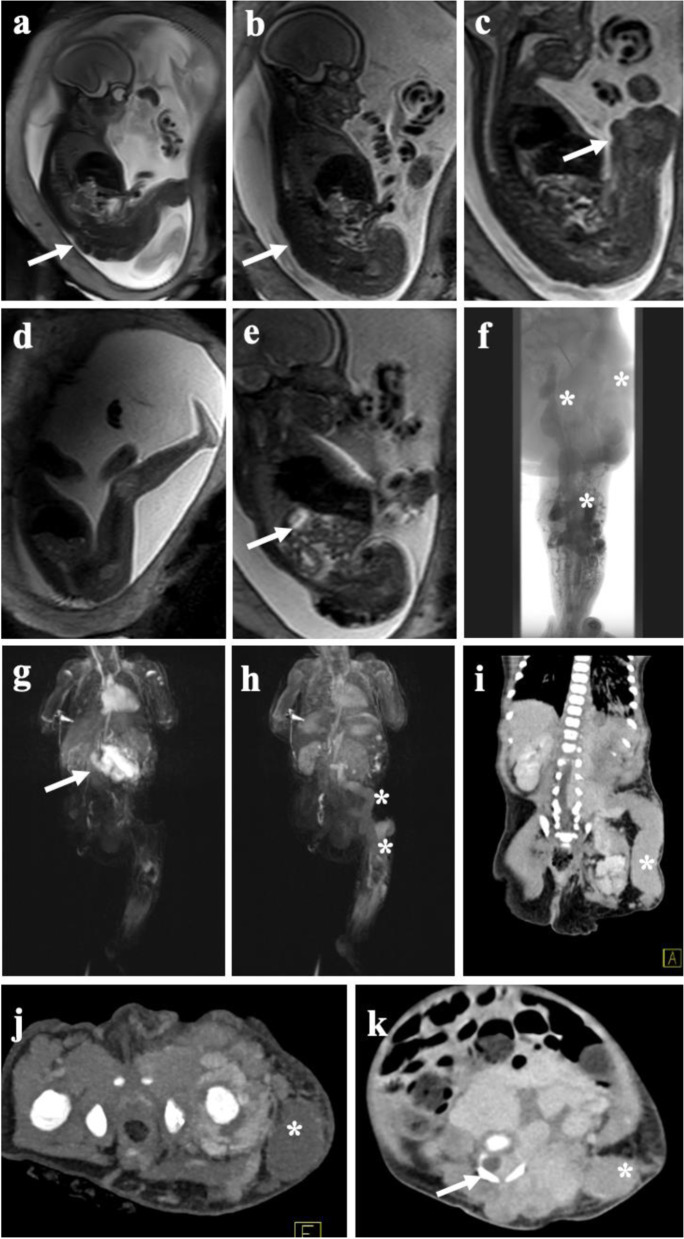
Fetal MRI and post-partum MRI/CT of complex vascular malformation. Fetal MRI using dedicated T2-weighted sequences reveal extensive venous malformation along the entire left leg (a) extending along the pelvis and lower back (b). c Large venous channel (arrow) raised suspicion of a large persistent marginal vein. d Healthy right leg in comparison. e Extension of the malformation in the retroperitoneal space. f Post-natal phlebography depicting large marginal vein together with extensive venous malformation of the lower and upper leg with extension into the pelvis (asterisk). Large blood volumes trapped in the marginal vein induced a localized consumptive coagulopathy in the child requiring immediate anticoagulation upon delivery. g Postnatal MRI revealing early arterial filling of an additional mesenteric arteriovenous malformation in the same patient, being asymptomatic at time of diagnosis. The course of the marginal vein, as depicted on MRI (h) and CT (i) showing large venous drainage into the pelvis (asterisk). j Transverse CT image in the arterial phase demonstrating multiple AV fistula paraspinal and along the left hip joint (arrows) together with the not yet contrasted marginal vein (asterisk). k Venous phase CT depicting the extension of intraabdominal, retroperitoneal, and paraspinal venous cavities with infiltration of the spinal canal (arrow) by the large slow-flow malformation, at time with contrast in the large marginal vein (asterisk). The large venous malformations required multiple rounds of image-guided embolization followed by stepwise surgery of the marginal vein
